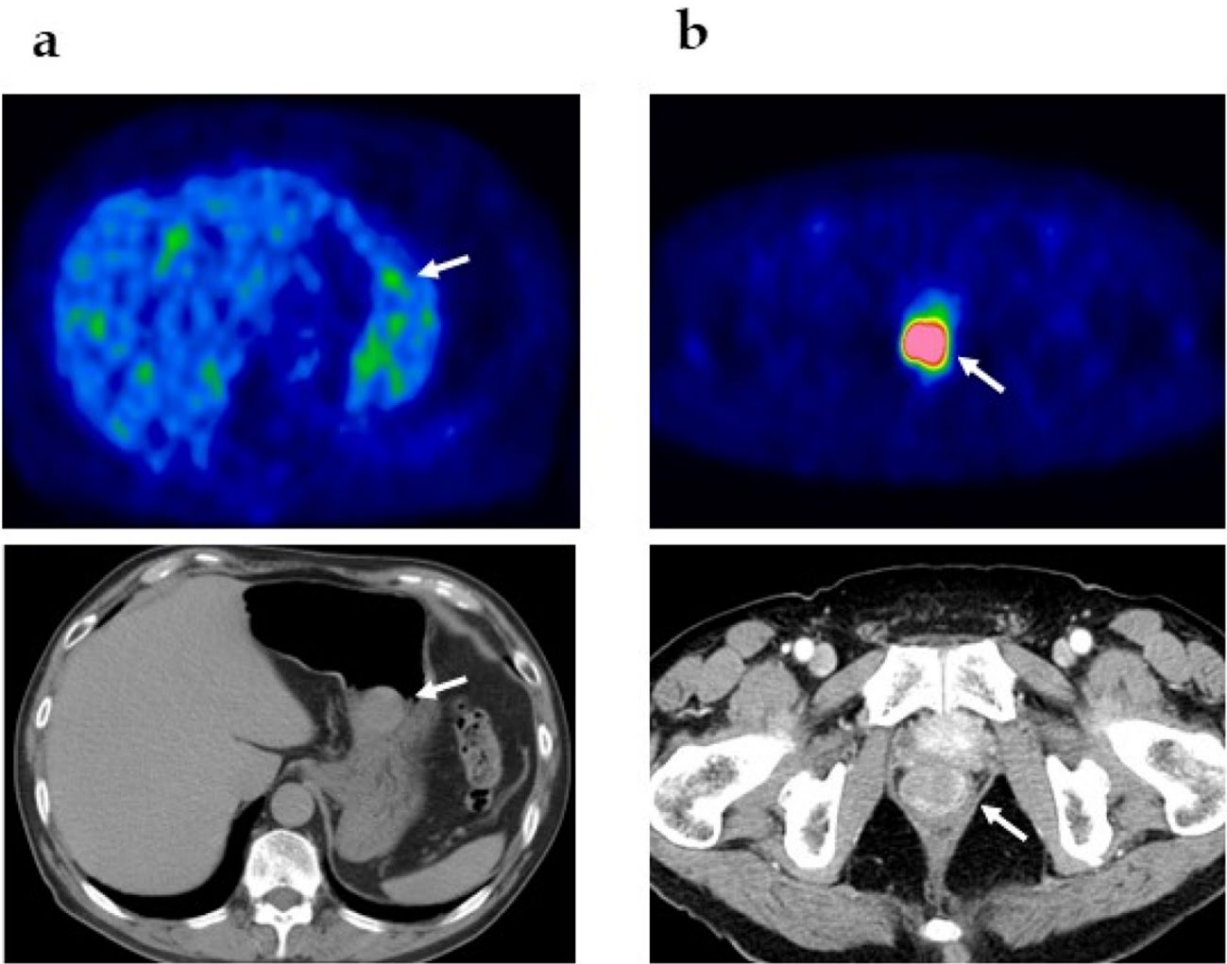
Representative cases. (a) Low-risk case in the stomach with a tumor size of 3.3 cm, mitosis 3/HPF, and SUVmax 4.58. (b) High-risk case in the rectum with a tumor size of 3.0 cm, mitosis 26/HPF, and SUVmax 20.68. Arrows indicate tumors.
